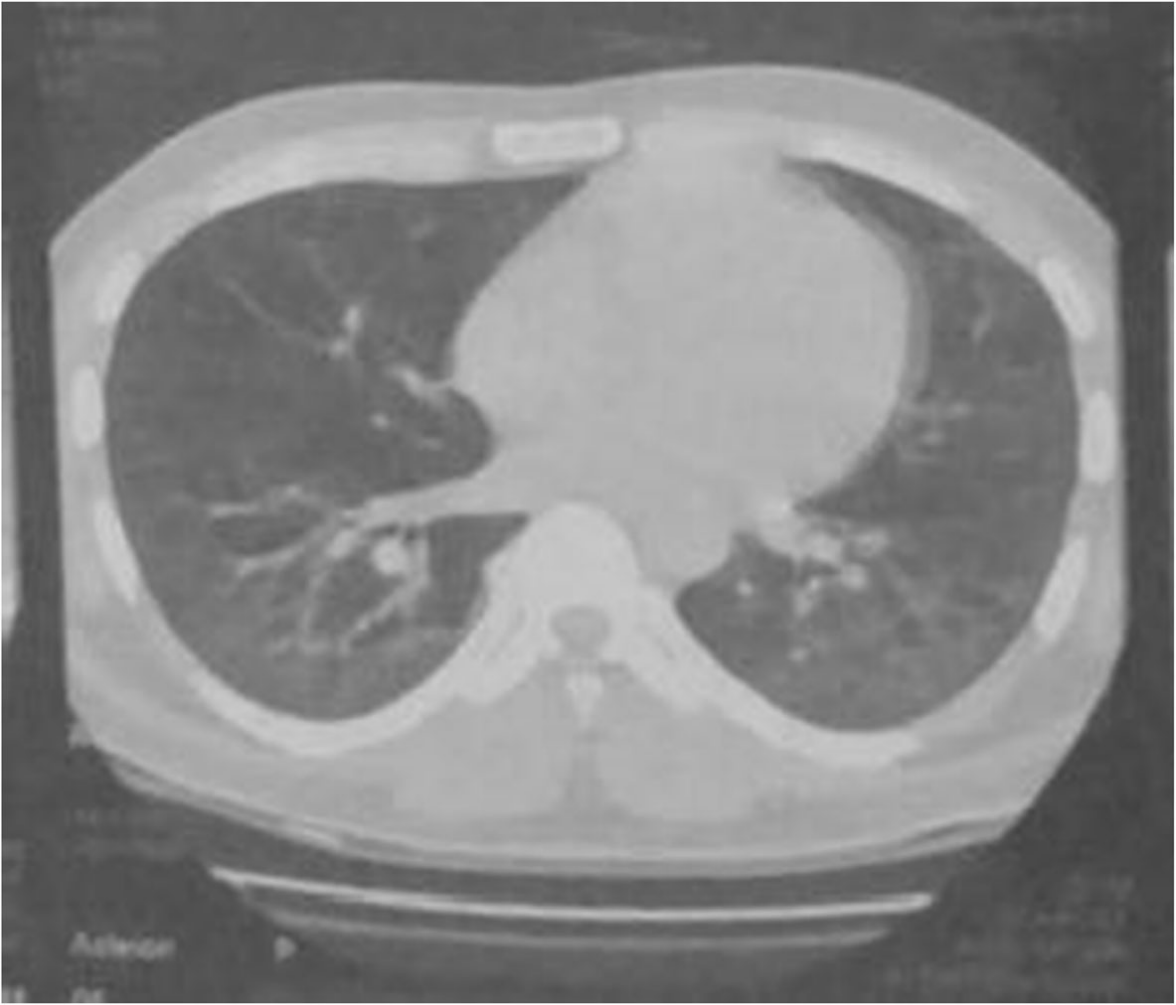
CT scan showing features suggestive of Interstitial Lumg Disease (ILD)
